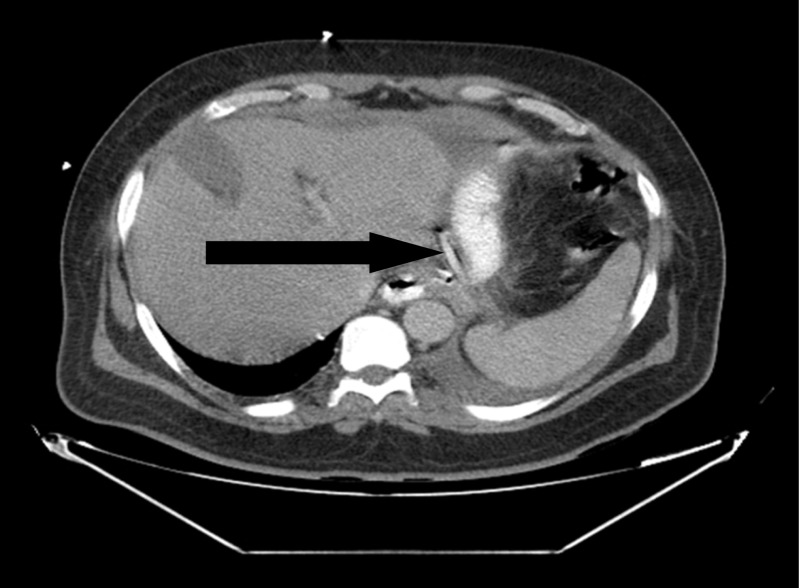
CT scan showing contrast in the hemovac tube (tiny leak of contrast from the 1st fistulo-jejunostomy)
